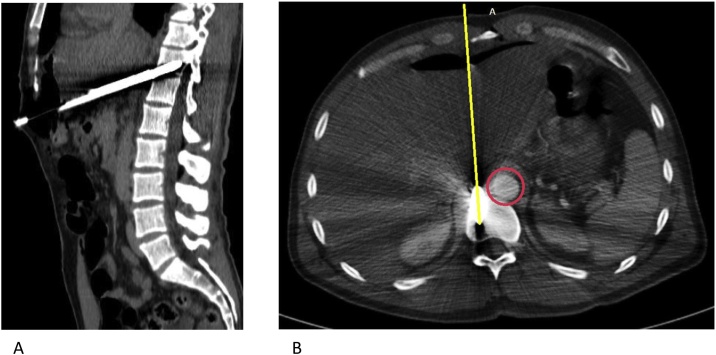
Abdominal CT (A: sagittal, B: frontal) showed the harpoon (yellow line) transfixing the 3rd hepatic segment, crossing the inter aorto-caval space (Aorta in red), and ending at the D11’s vertebral body.
